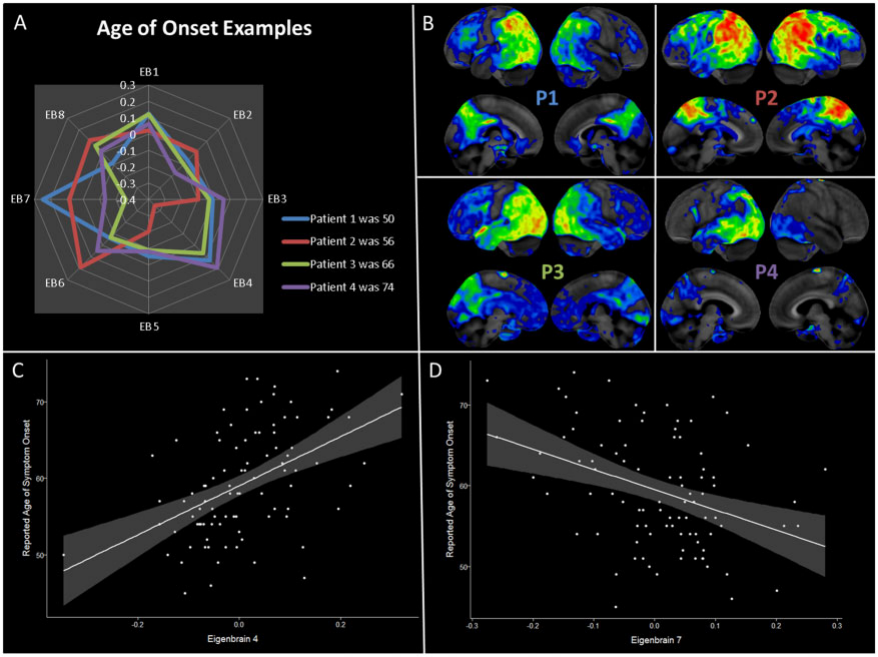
Relationship between age of onset and eigenbrain weights. Age of reported symptom onset was significant across multiple eigenbrain vectors (with over 50% of variance explained by eigenbrain vectors), but this association was strongest in EB4 and EB7. (A) Four individuals with a wide variation in age are mapped onto the eight primary eigenbrains. Positive weighting to EB7 and/or negative weighting to EB4 are associated with younger age of symptom onset (Patient 1 and 2). Positive weighting to EB4 and/or negative weighting to EB7 are associated with older age of symptom onset (Patient 3 and 4). (B) The four individual FDG PET scans are shown in the same anatomical orientation as Fig. 2. (C) Eigenbrain 4 has a significant association with reported age of symptom onset (*r*^2^ = 0.23, *t*-value 5.2, *P* < 0.001). (D) Eigenbrain 7 had an opposite but significant association with reported age of symptom onset (*r*^2^ = 0.15, *t*-value −3.8, *P* < 0.001).
